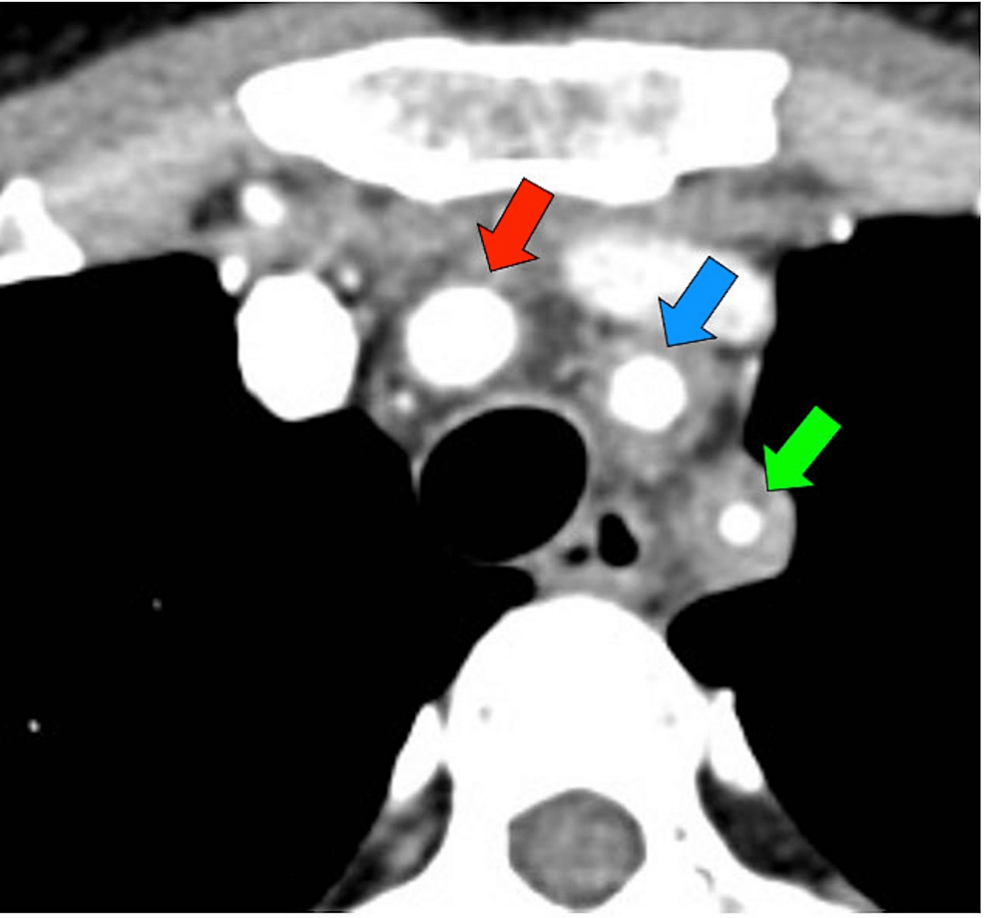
Contrast-enhanced computed tomography scan (axial view) exhibiting wall thickening in the brachial (red arrow), left common carotid (blue arrow), and left subclavian (green arrow) arteries.
